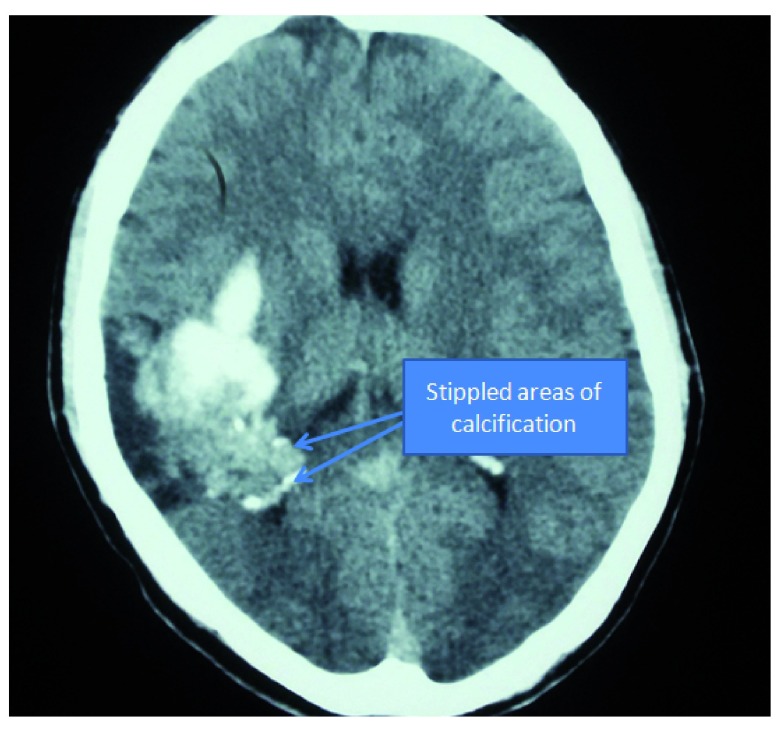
CT showing presence of hyperdense lesion in the right parietal region with stippled calcification on the periphery.
